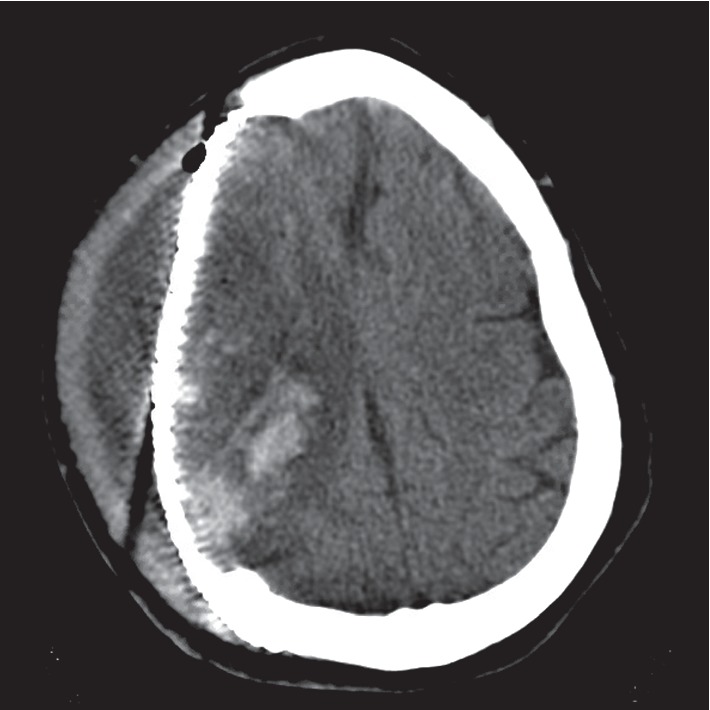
Head CT revealing an 18 mm subgaleal fluid collection overlying the duraplasty.
